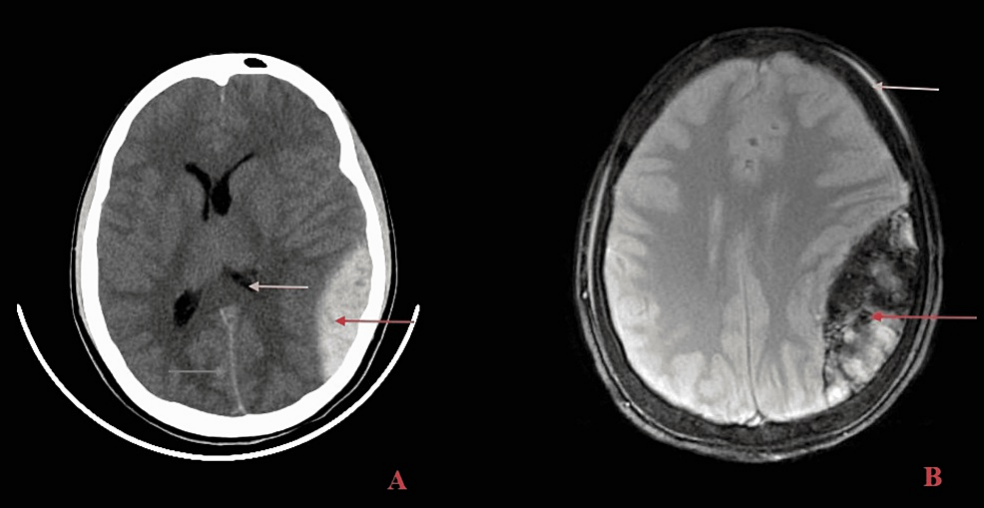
A. CT brain axial section showing a biconvex hematoma, suggestive of acute EDH (red arrow), with midline shift to the right (blue arrow), and effacement of the left lateral ventricle (yellow arrow). B. MRI brain axial section showing acute and ongoing EDH (red arrow). Diffuse cerebral edema is present, and mild scalp contusion is noted over the left parietal region (yellow arrow). CT - computed tomography; EDH - epidural hematoma; MRI - magnetic resonance imaging
